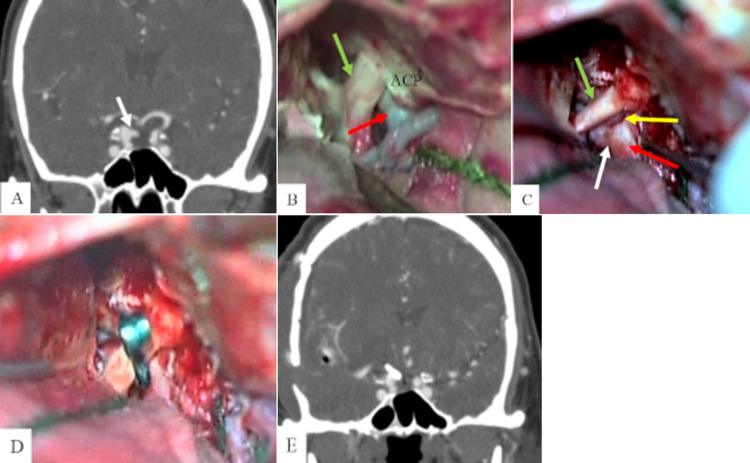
Tailored intradural AC (partial resection) (A) Preoperative coronal CT angiography reveals a 7-mm saccular aneurysm of the ICA, ophthalmic segment, on the right side (white arrow). (B) The intradural view shows the optic nerve (green arrow), ICA (red arrow), and ACP, but not the aneurysm. The aneurysm and the ophthalmic artery cannot be fully visualized due to the ACP. (C) The aneurysm and its neck (white arrow), as well as the ophthalmic artery (yellow arrow), are clearly identified after partial AC (optic canal unroofing and ACP tip osteotomy). (D) The surgical clip is successfully applied and the aneurysm is occluded, with good control of the ophthalmic artery. (E) Postoperative coronal CT angiography shows total occlusion of the aneurysm. ACP, anterior clinoid process; ICA, internal carotid artery; AC: anterior clinoidectomy
